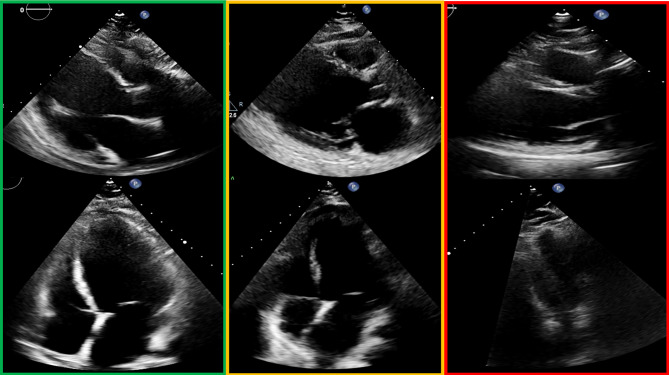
Representative transthoracic images at various stages of Duchenne muscular dystrophy disease progression. Top panel - Parasternal long axis (PLAx); Lower panel –Apical four-chamber (A4Ch); Left panel (green): 17-year-old patient at time of transition from paediatric care. Not yet requiring non-invasive ventilation; Middle panel (orange): 19-year-old patient with previous corrective spinal surgery for scoliosis. Not yet requiring non-invasive ventilation; Right panel (red): 36-year-old patient with prior scoliosis surgery. Established on non-invasive ventilation. The images demonstrate the progressive deterioration of image quality in the setting of disease progression and the establishment of non-invasive ventilation
